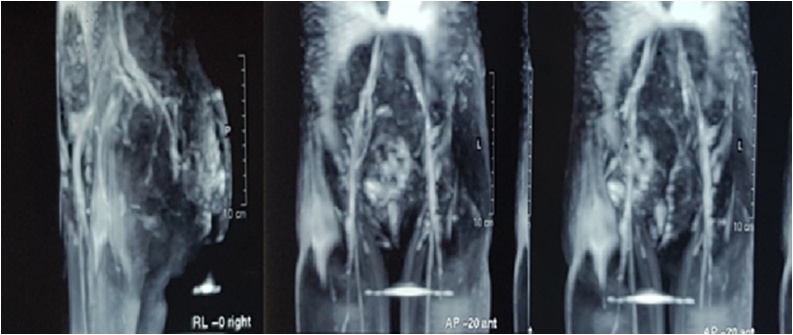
Post-operative MRI showing the remnant small nidus of the lesion.
